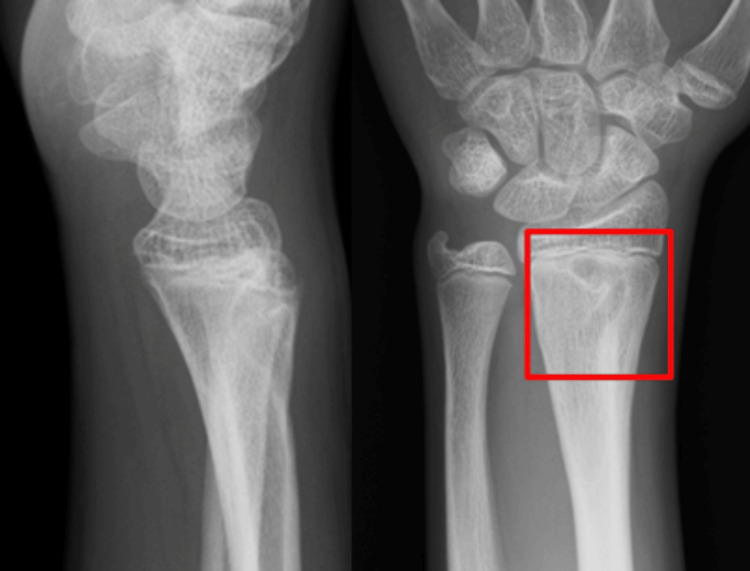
X-ray showing a radiolucent area in the distal radius (red box)
